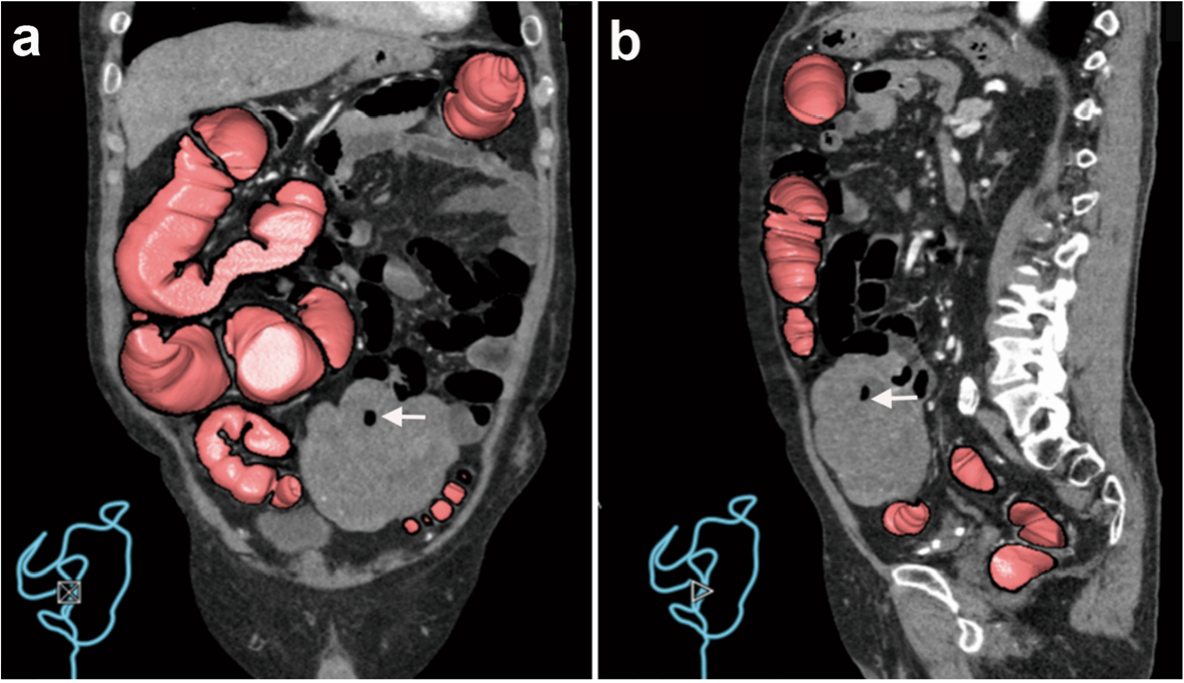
Virtual endoscopic images with multi-planar reconstruction on computed tomography colonography. a Coronal and (b) sagittal planes show the huge tumor originating from the small intestine and not from the colon. The bowel tract (in pink) represents the colorectum. The arrows represent an air bubble in the tumor
